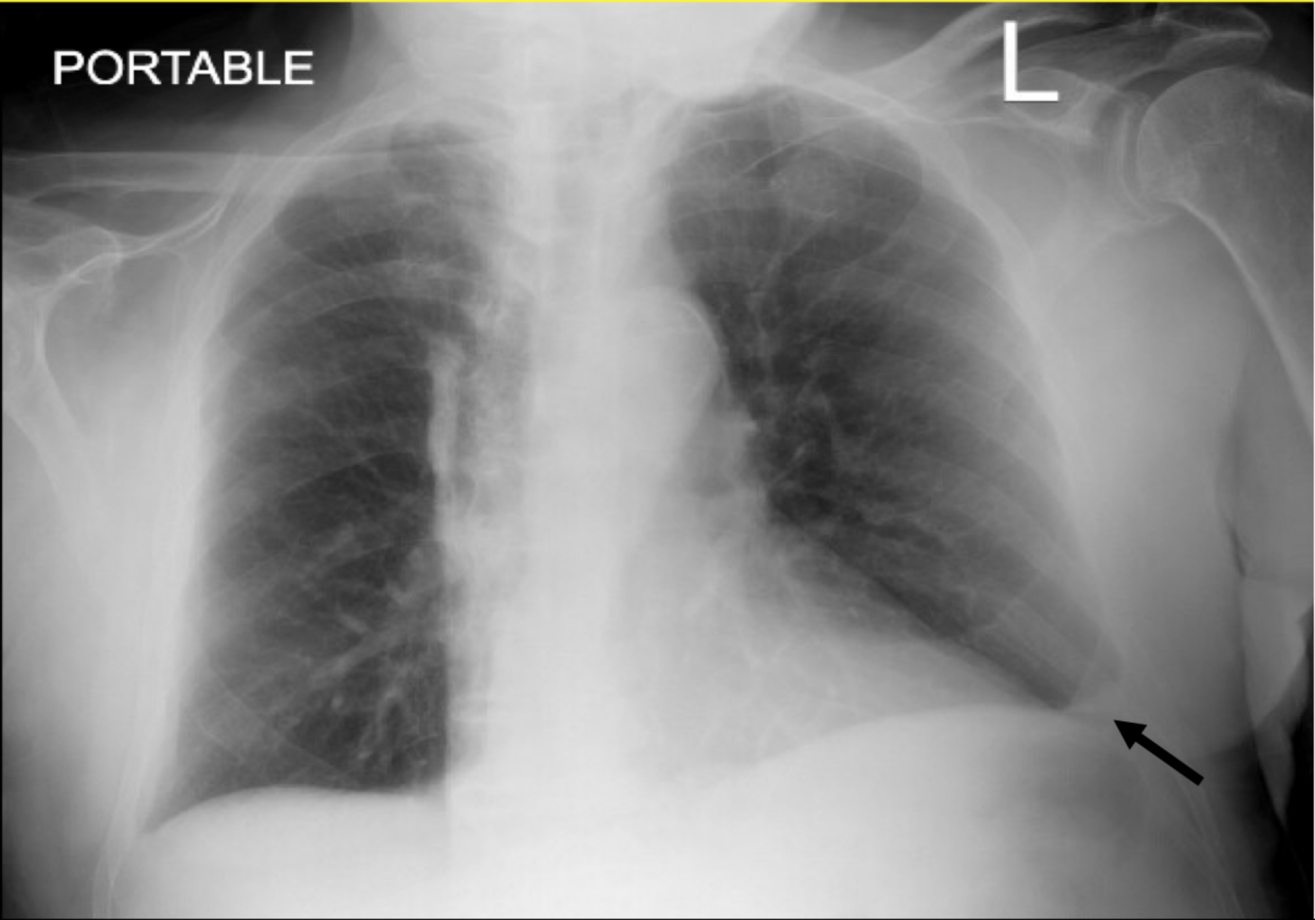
Chest X-ray (CXR) showed left small pleural effusion
